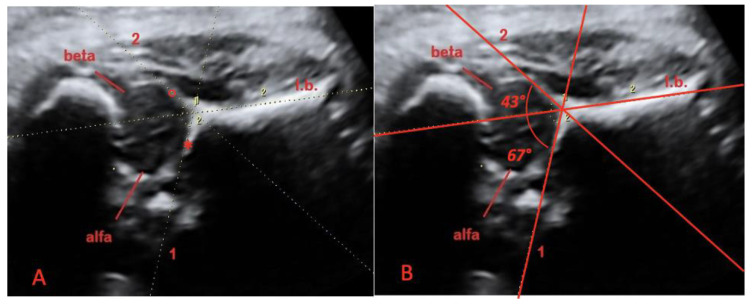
(A) Midsagittal plane of the coxofemoral joint, obtained with 3D ultrasound, in a fetus at 37 weeks of gestation: the “Y” sign. The iliac bone (I.b.), the labrum acetabular (°) and the lower edge of ossified ilium (*). (B) Midsagittal plane of coxofemoral joint, obtained with 3D ultrasound, in a fetus at 37 weeks of gestation: the “Y” sign. The alpha angle (“bone angle”) is the angle formed by a line tangential to the horizontal iliac wing (I.b.) and a line tangential to bone roof (*). The beta angle (“cartilage angle”) is the angle formed by a line tangential to the horizontal iliac wing (I.b.) and a line tangential to cartilaginous roof (°).
